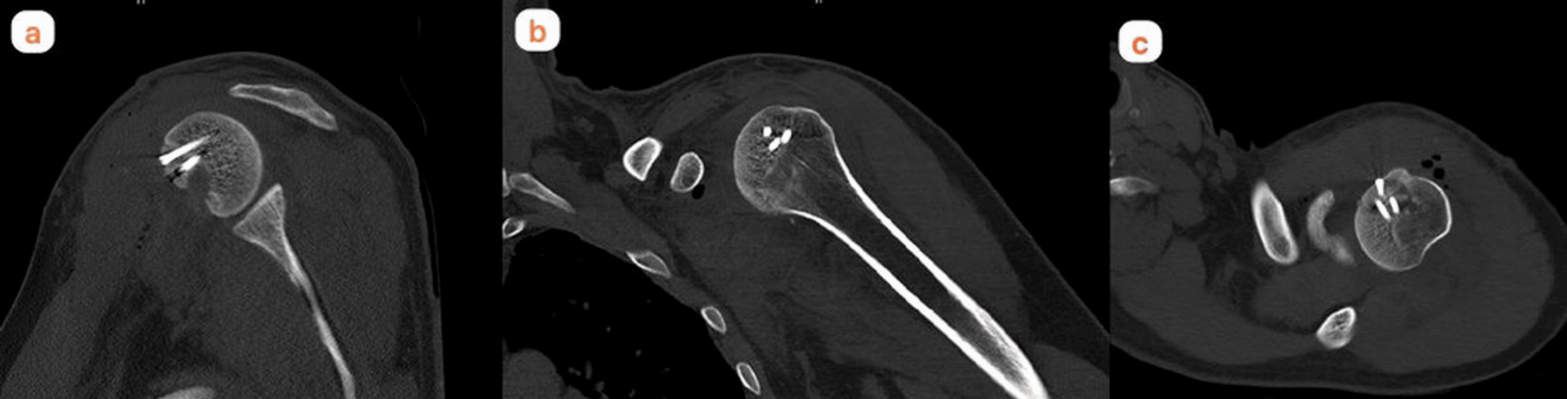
Sagittal (a), coronal (b) and axial (c) sections of the patient's left shoulder CT imaging at the last follow-up (Patient 1)
